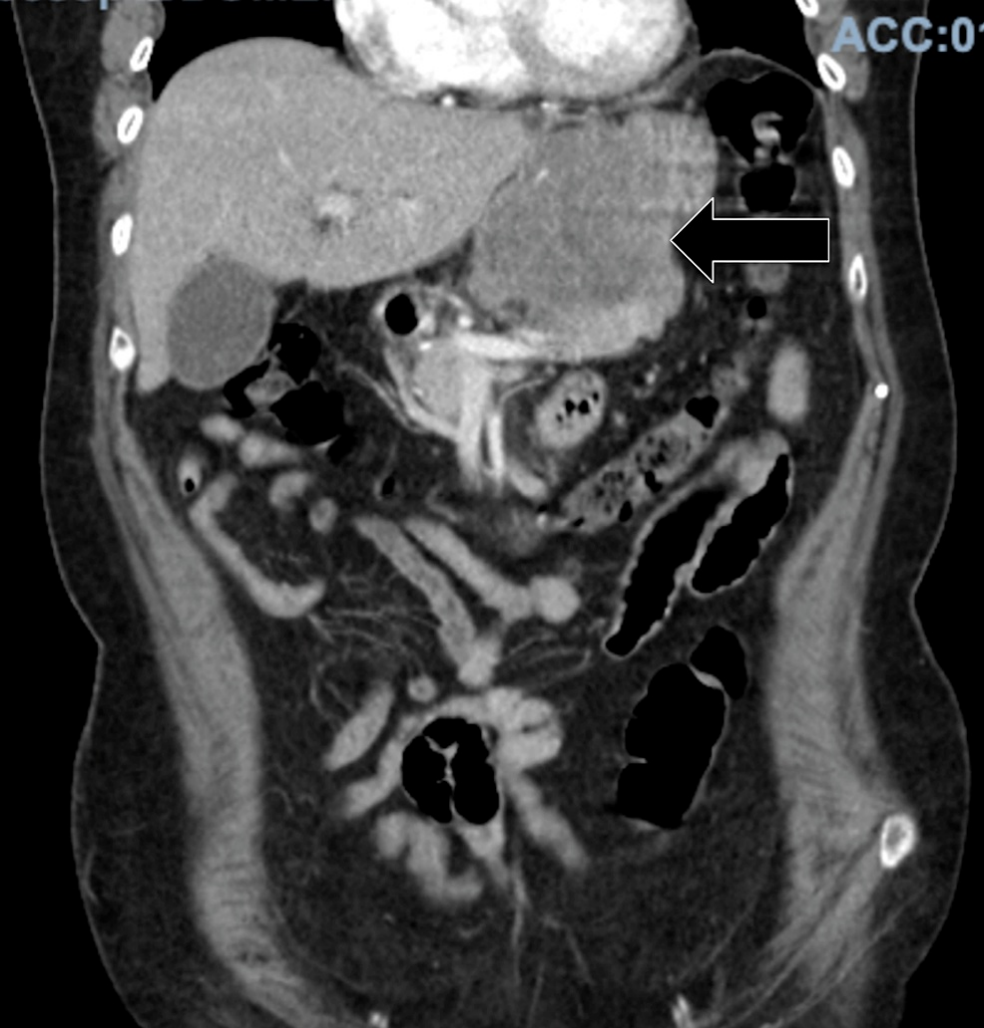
CT scan of the abdomen performed upon admission The image shows a large, 7.6 x 5.9 x 6.2-cm intraabdominal mass arising from the stomach and extending to the lesser sac and the central crus of the diaphragm (arrow) CT: computed tomography
